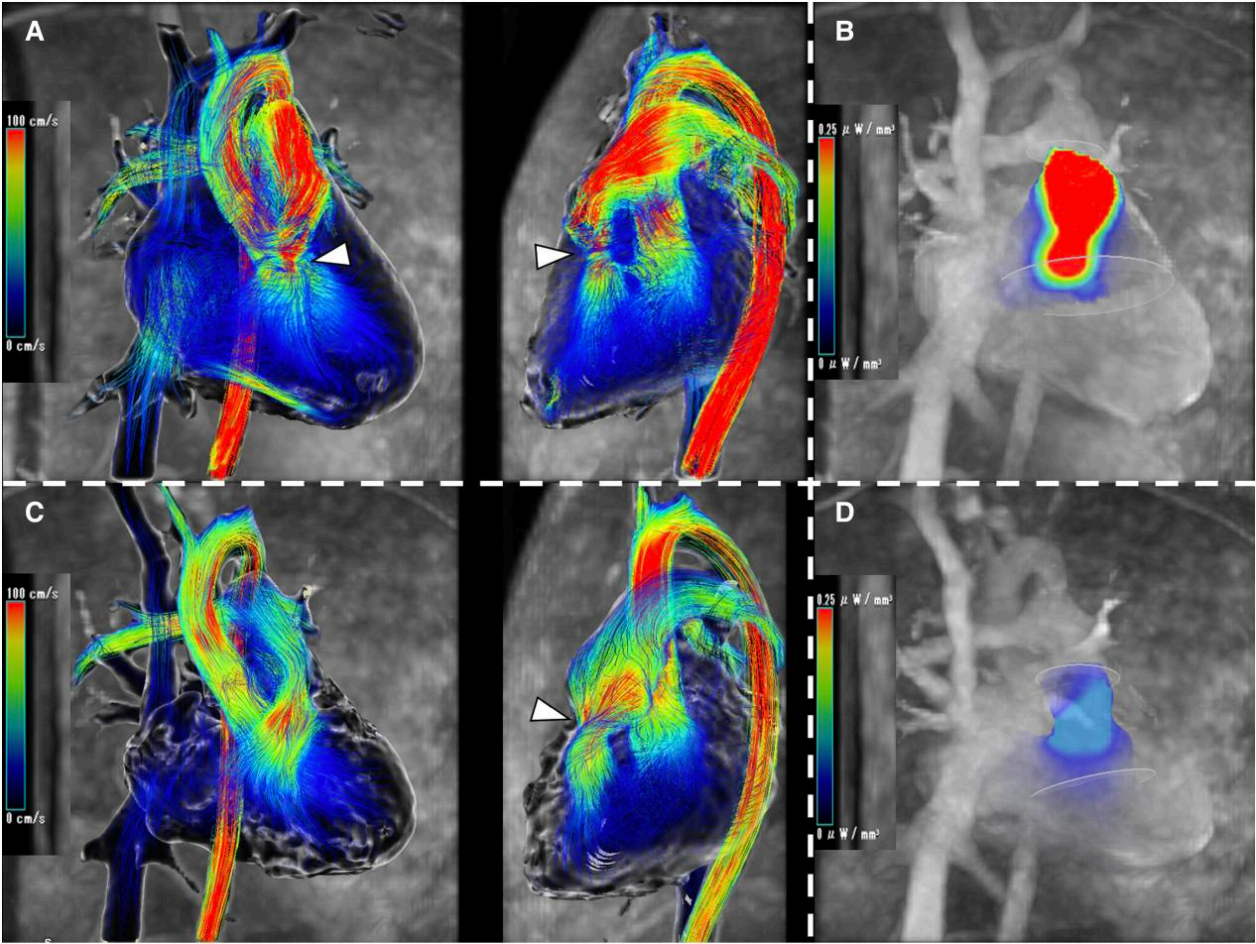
An 18-year-old male was diagnosed with a perimembranous ventricular septal defect (VSD) at birth and followed up. At 10 years old, the defect size was 3 mm, echocardiography showed right ventricular (RV) outflow obstruction (continuous wave 2 m/s), and he developed a double-chambered right ventricle (DCRV). At 18 years old, blood flow was 5 m/s by echocardiography and the pressure gradient (PG) was 37 mmHg by cardiac catheterization under sedation, and he wanted treatment. The thickened muscle bundle resection and VSD patch closure were done, and post-operative measurements with a Swan–Ganz catheter showed that the PG had disappeared. Echocardiography also showed that blood flow improved to 1.4 m/s, and the obstruction was sufficiently released. 4D flow MRI showed significant helical flow before surgery (*Panel A*, Supplementary data online, *Video S1*). The energy loss (EL, highest/mean value) was 7.54/1.87 mW (EL 0.57/0.16 mW in a healthy subject) (*Panel B*). Post-operatively, the helical flow was reduced but remained (*Panel C*, Supplementary data online, *Video S2*), and the EL was also lower at 1.45/0.42 mW, but higher than normal (*Panel D*). One month later, blood flow has accelerated to 2.3 m/s. The patient is being carefully monitored. The RV outflow obstruction is an ongoing process that may not depend only on PG, but cellular apoptosis and/or proliferation may be subjected to intracavitary blood vortices and surgery should look at imaging in pre-/post-surgical planning. In patients with DCRV, if the helical flow does not disappear after surgery, careful follow-up is needed to watch for recurrence.
